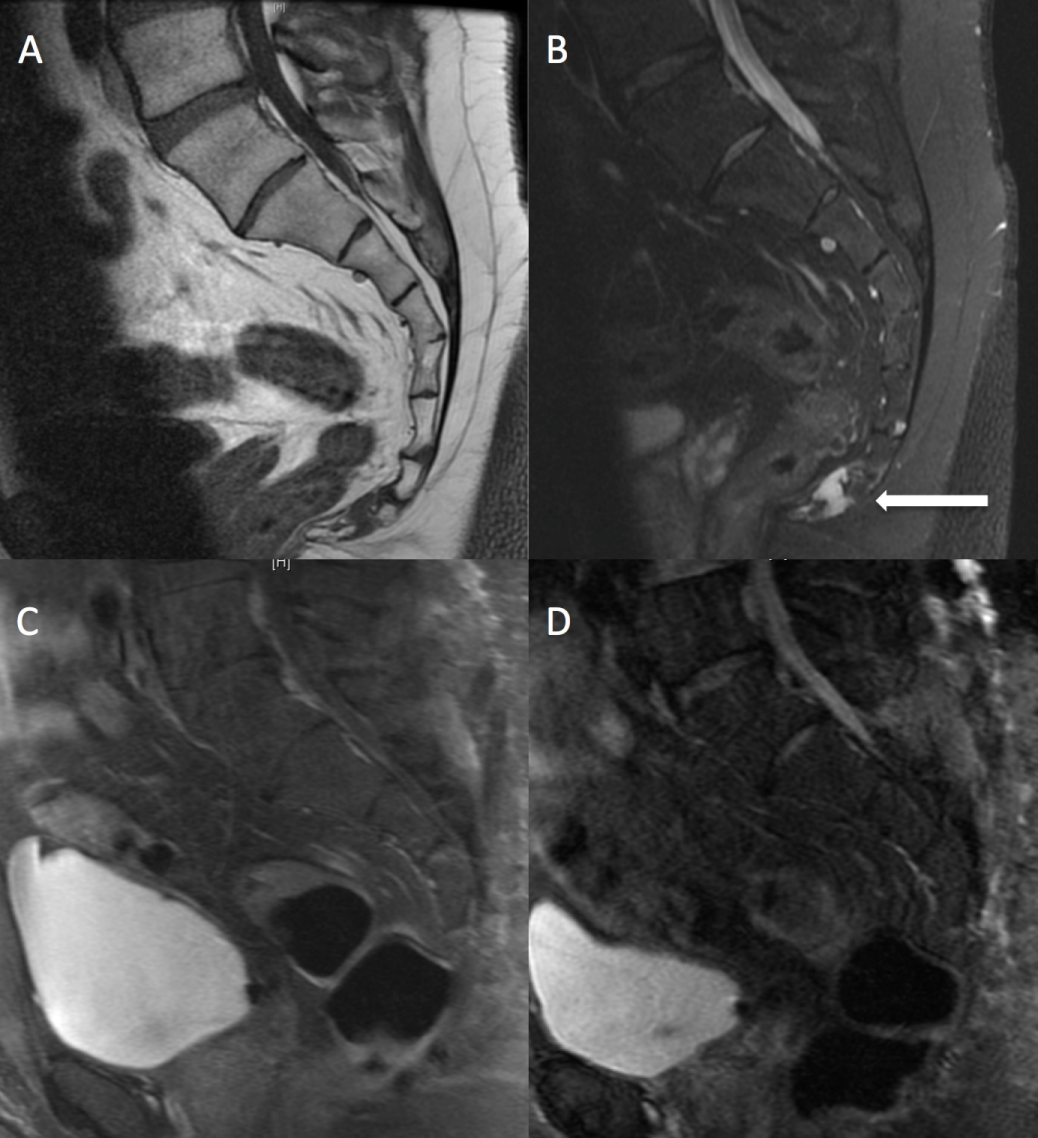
Patient 1 - Preoperative and postoperative imaging Preoperative MRI (A, B) demonstrates T2 intense well-circumscribed sacrococcygeal mass approximately 3 x 2 x 2 cm in size involving the S4 nerve roots. Postoperative MRI (C, D) shows en bloc total resection of tumor.
